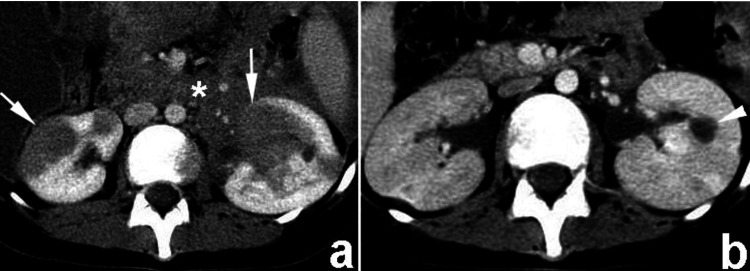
During the nephrographic phase, contrast-enhanced CT (CECT) imaging was conducted both before (a) and after (b) six months of treatment. The scans reveal the presence of a significant infiltrative mass of soft tissue, denoted by the asterisk, indicative of lymphoblastic lymphoma. According to its infiltrative pattern, the mass extends into the retroperitoneum and invades the renal sinus and parenchyma, as highlighted by the arrows. Following six months of treatment, a subsequent CT scan demonstrates complete resolution of both the renal and retroperitoneal tumors. Notably, attention is drawn to the identification of a small cyst located on the left kidney, denoted by the arrowhead. This figure is a republished image, permission was obtained from the original publisher, https://epos.myesr.org/poster/esr/ecr2018/C-2802
